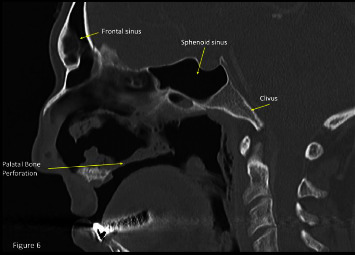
The sagittal images of the CT scan revealed bony and cartilaginous destruction of the nasal septum and the palate. The rhinopharyngeal mucosa is eroded with occipital bone clivus exposition.
